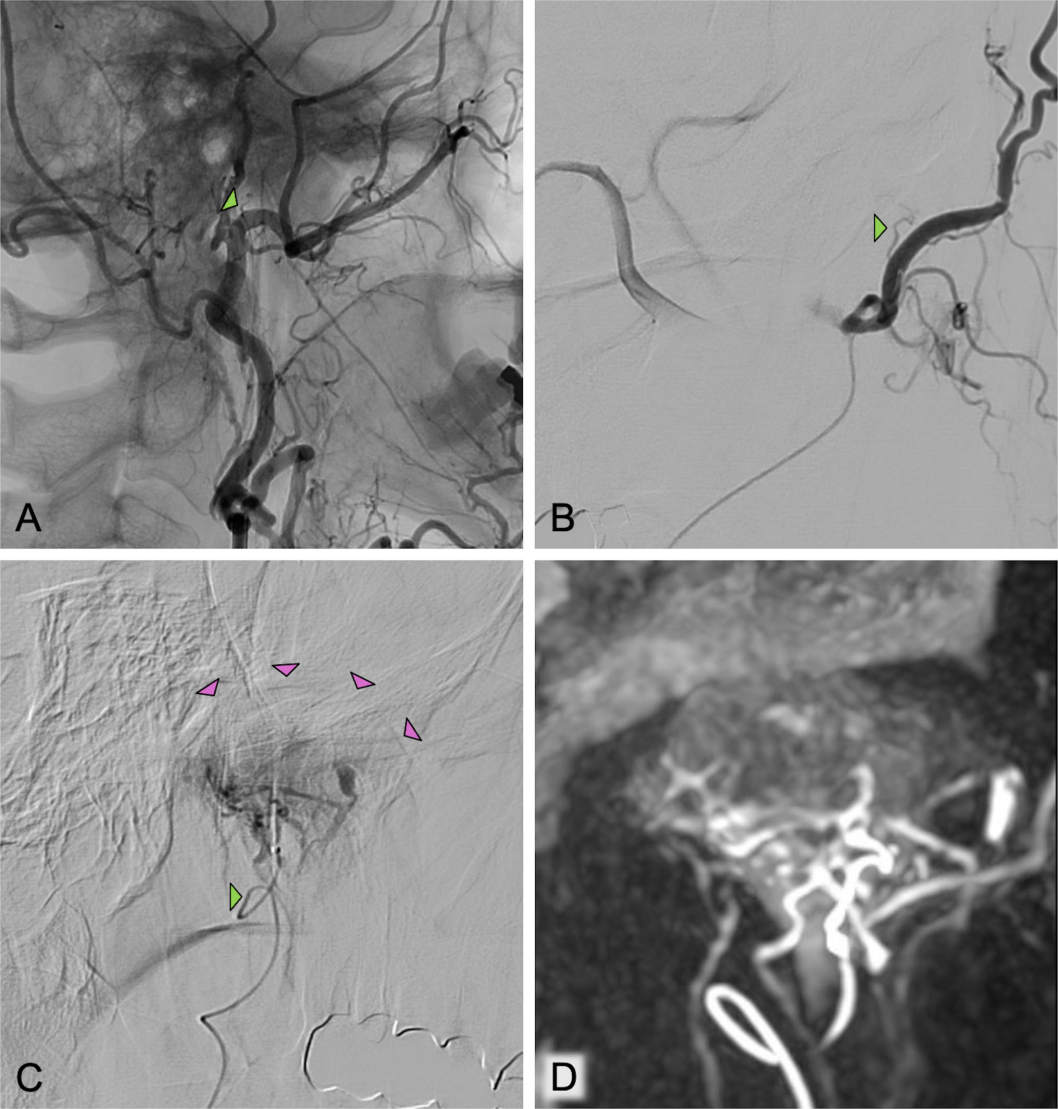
A. Angiogram from the ECA to identify the TMJ-feeding arteries and to evaluate for potential extra- intracranial anastomoses. B. Selective angiogram of the IMAX with an early origin of the dominant TMJ-feeding artery (green arrows, respectively) C. Superselective angiogram of the TMJ-feeding artery revealing the OA-typical hyperemic blush. The purple arrows mark the joint space confirming correct positioning on the angiogram. D. MIP-CBCT reconstruction of the 3D angiogram from the superselective tip position of the microcatheter revealing a net of arteries formed around the TMJ (rete articulare mandibula). TMJ temporomandibular joint; ECA external carotid artery; IMAX internal maxillary artery; MIP maximum intensity projection; CBCT cone-beam CT
